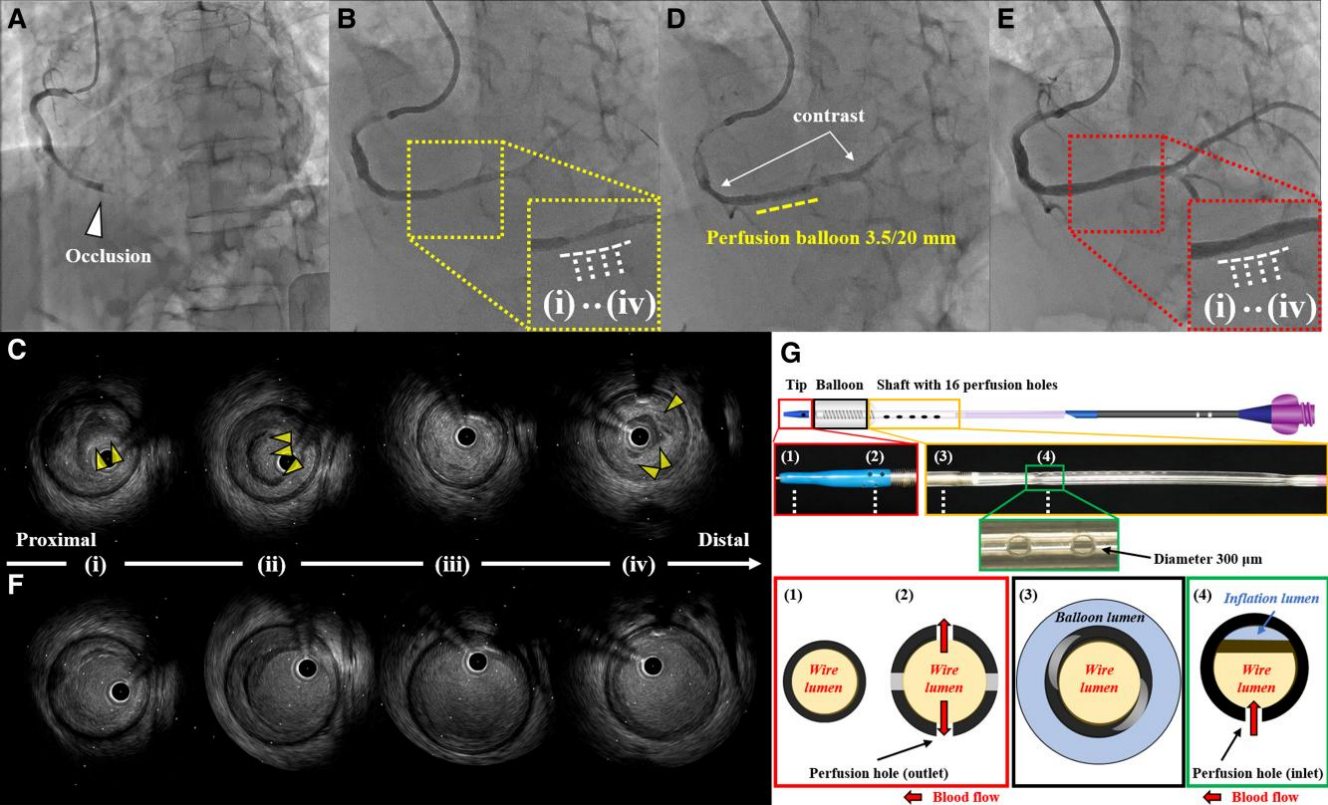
(*A*) Initial and (*B*) post-thromboaspiration coronary angiography. (*C*) Initial intravascular ultrasound showing residual thrombus and plaque in each lesion (i–iv) visible on *B*. (*D*) Post-perfusion balloon angioplasty and final (*E*) coronary angiography. (*F*) Final intravascular ultrasound images showing optimal lumen enlargement. Arrowheads indicate the residual thrombus. (*G*) Structure of a perfusion balloon, which can perfuse blood or intracoronary-infused drugs through wire lumen via multiple side holes in the shaft.
